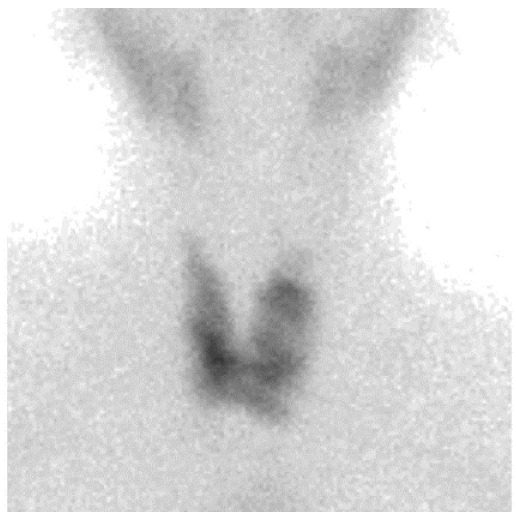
Thyroid scintigraphy with TC-99 74 MBq iv: Glandular parenchyma of the thyroid is not homogeneous, due to the presence of various hypocaptic areas in the context of both lobes, of which the two largest were located one in the right upper lobe and the other in the middle third and at the base of the left lobe.
